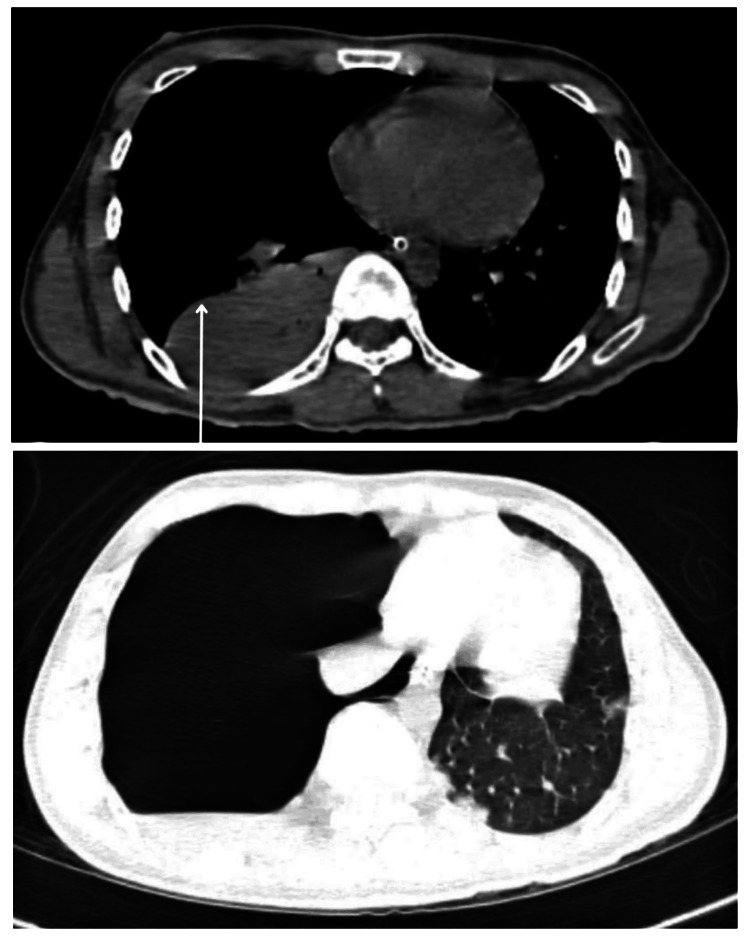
HRCT Thorax revealing right-sided tension pneumothorax along with areas of consolidation remarkably noted superior segment of left lower lobe HRCT- High-resolution computed tomography
